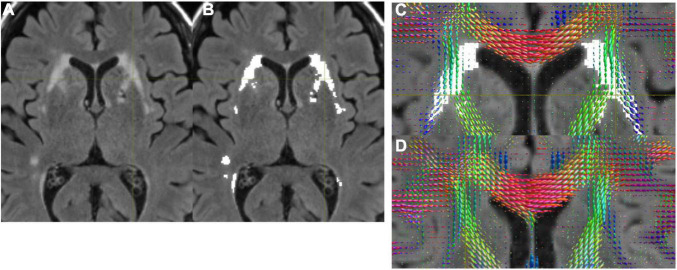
(A) A FLAIR image. (B) WMH was successfully segmented by the automated program. (C) FODs were overlaid on FLAIR with WMH. Altered FODs demonstrated in the WMH regions, compared to similar regions of a subject without WMH (D).
